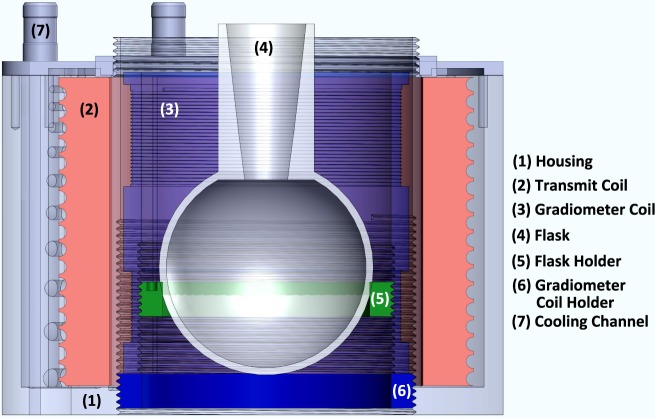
The field generator of INSPECT consisting of (1) the main body which houses the transmitting coil, (2) the transmitting coil, (3) the gradiometer coil, (4) the flask for synthesis, (5) the flask holder, (6) the gradiometer coil holder, which could be mechanically adjusted to attain the maximum sensitivity of the particle signal and attenuation of the excitation frequency, and (7) the cooling channel for pumping pressurized air in and around the transmitting coil and the gradiometer coil.
